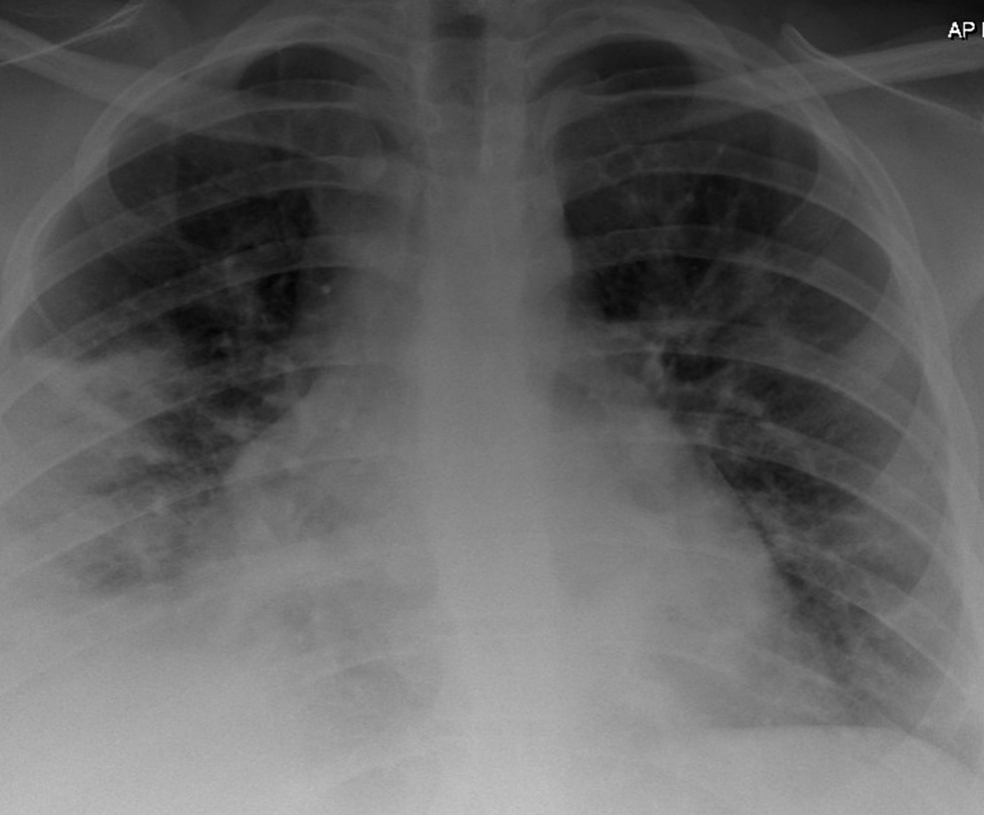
Chest X-ray showing COVID pneumonia
